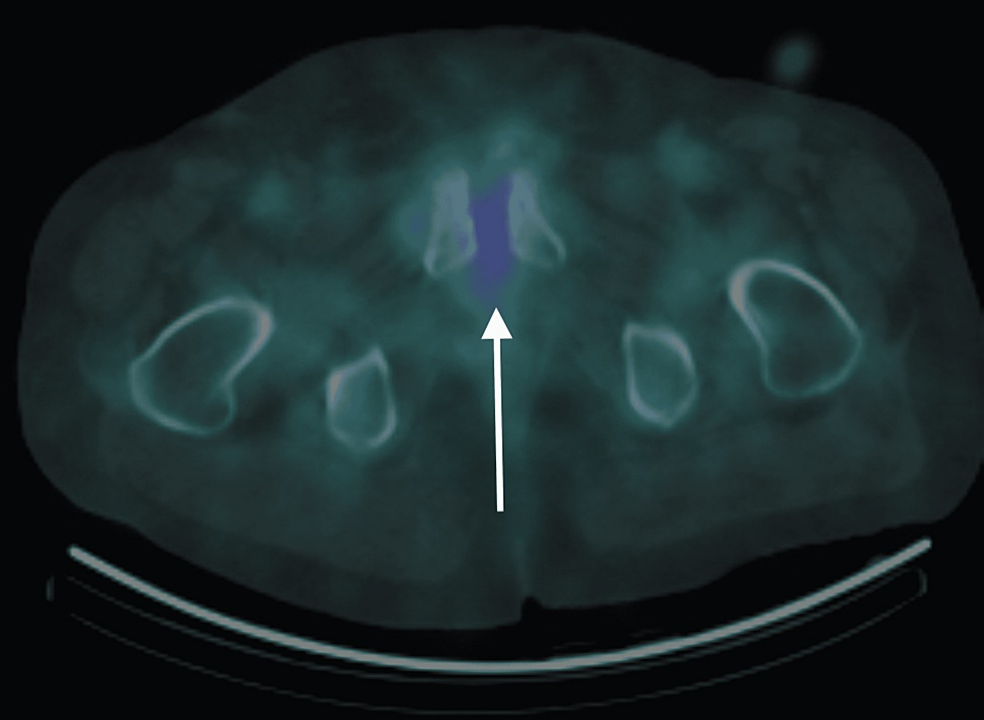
Gallium scan SPECT/CT. Axial SPECT/CT gallium scan (48 hours post-gallium 67 citrate injection) shows minimal uptake within the pubic symphysis in comparison to the bone scan done previously. This is consistent with treated osteomyelitis. SPECT/CT: single-photon emission computed tomography/computed tomography
